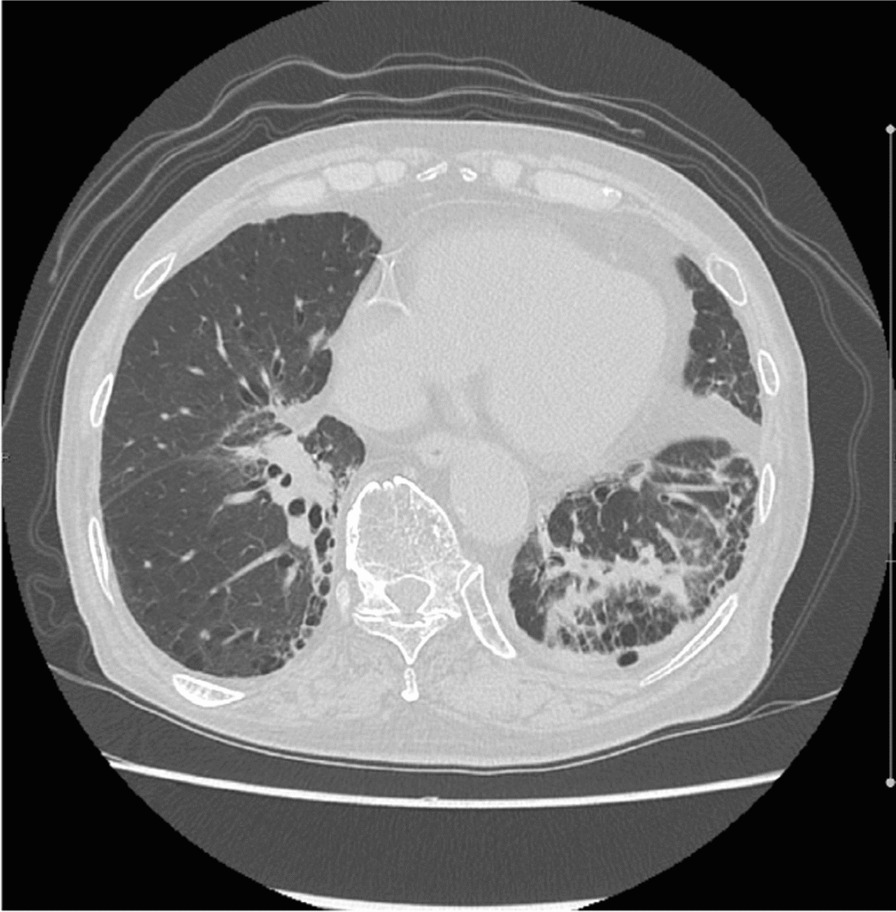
The most recent computed tomography scans. Computed tomography 37 months after the first stereotactic body radiation therapy and 11 months after the second stereotactic body radiation therapy
